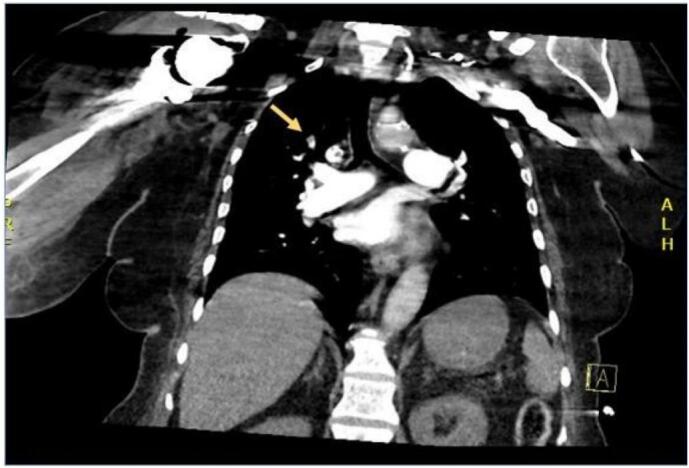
Spiral computed tomography showing right upper segmental lobe filling defect suggestive of pulmonary embolism.
